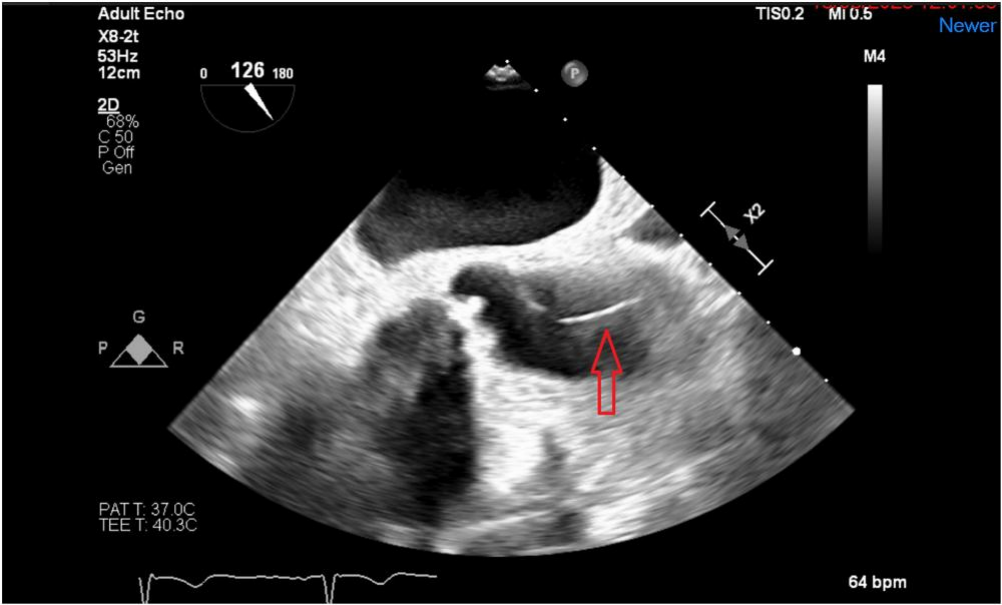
Trans-esophageal echocardiography.
